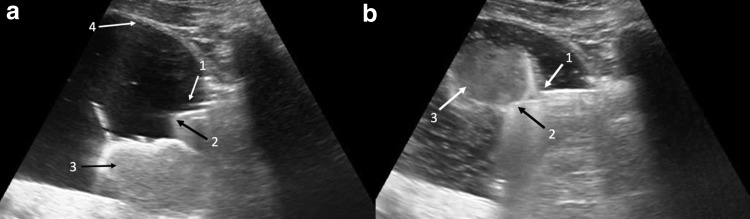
Ultrasonographic images of bladder, sagittal view. *Black* and *white arrows* with corresponding numbers used to label key structures. (a) The morcellator can be seen as it is introduced into the bladder. 1 = tip of nephroscope; 2 = tip of morcellator; 3 = dependent enucleated adenoma; 4 = bladder wall. (b) The morcellator is now imaged, positioned safely in the middle of the bladder, with the engaged adenoma being morcellated. 1 = tip of nephroscope; 2 = tip of morcellator; 3 = enucleated prostate adenoma engaged in morcellator.
